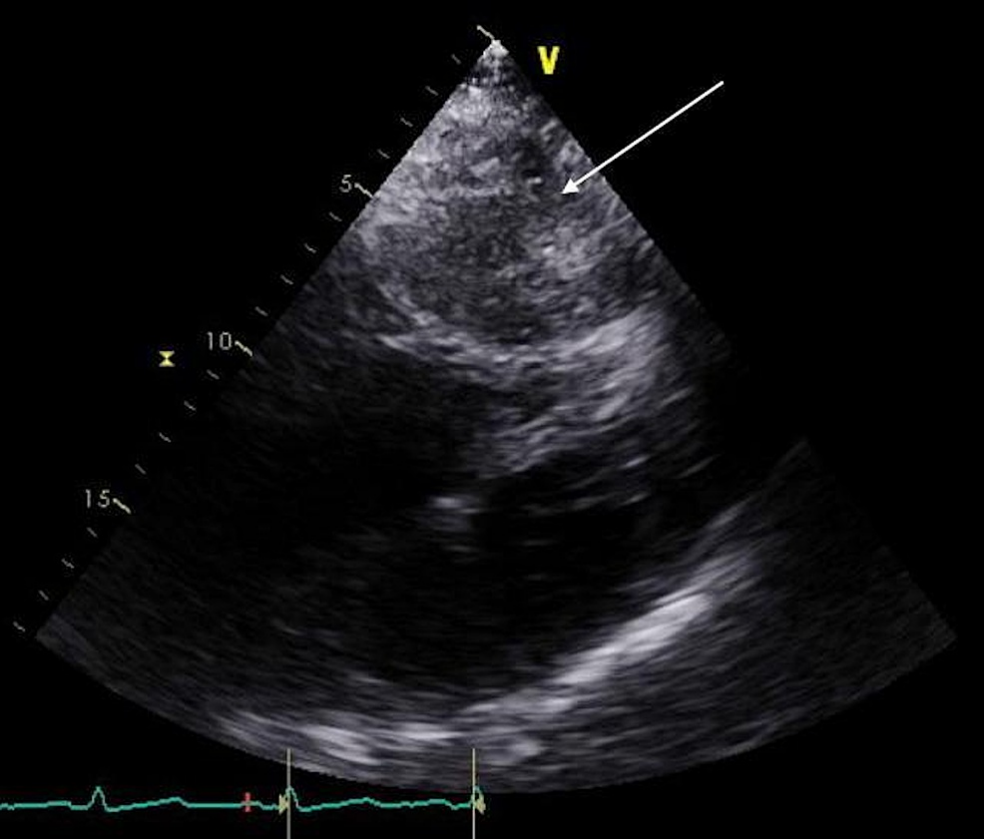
Subxiphoid view of TTE with visible hiatal hernia exhibiting compressive effect on left atrium and left ventricle (arrow). TTE: transthoracic echocardiogram
